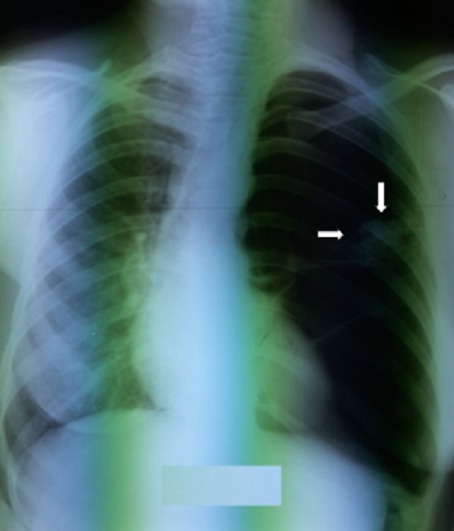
Radiographie thoracique à l’admission montrant le pneumothorax gauche avec l’opacité ronde
